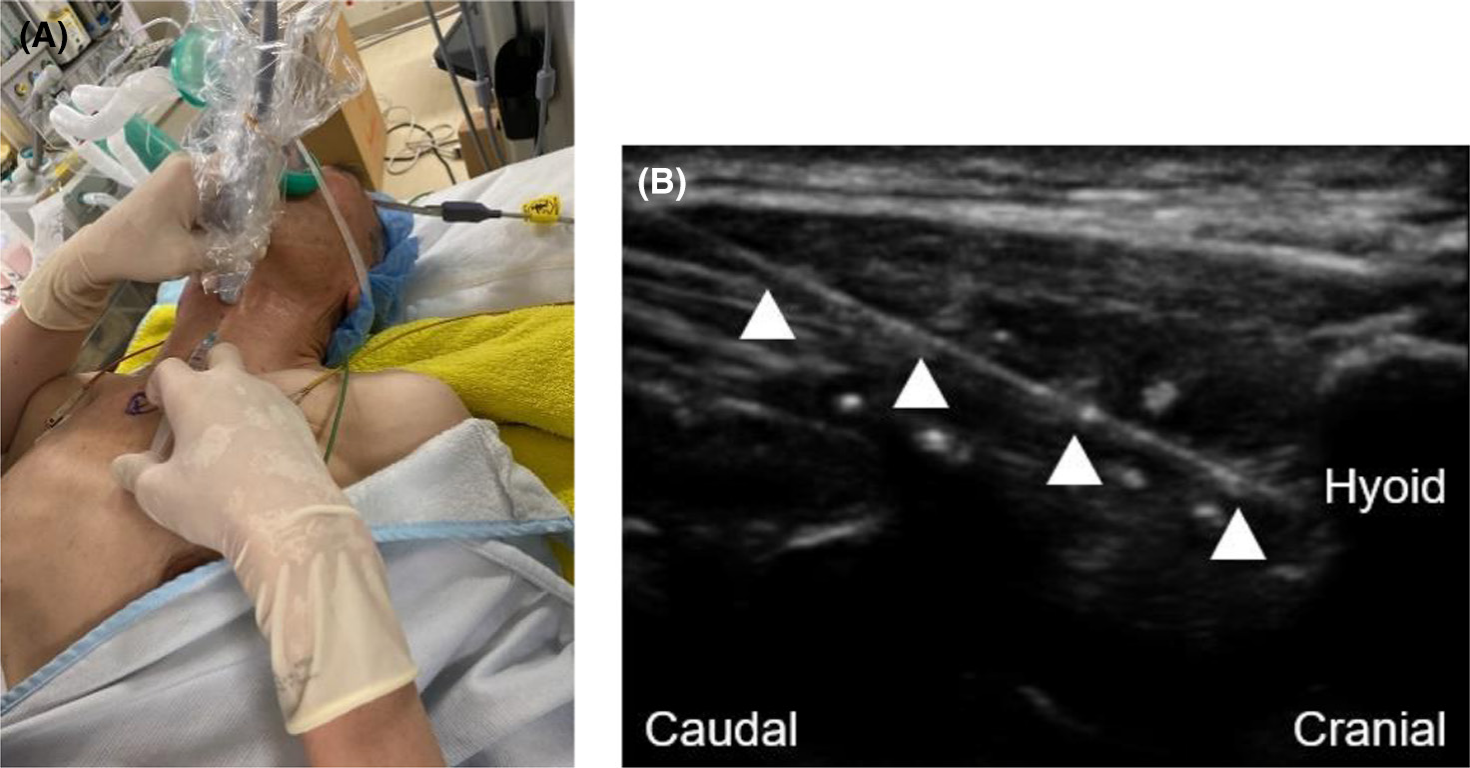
State at the superior laryngeal nerve block. Image of actual implementation of the superior laryngeal nerve block, (A) and a real‐time ultrasound image of the nerve block, (B) The superior laryngeal nerve block was performed in the sagittal plane under real‐time ultrasound guidance. The tip of the needle (indicated by the arrowheads) was positioned around the greater horn of the hyoid bone.
